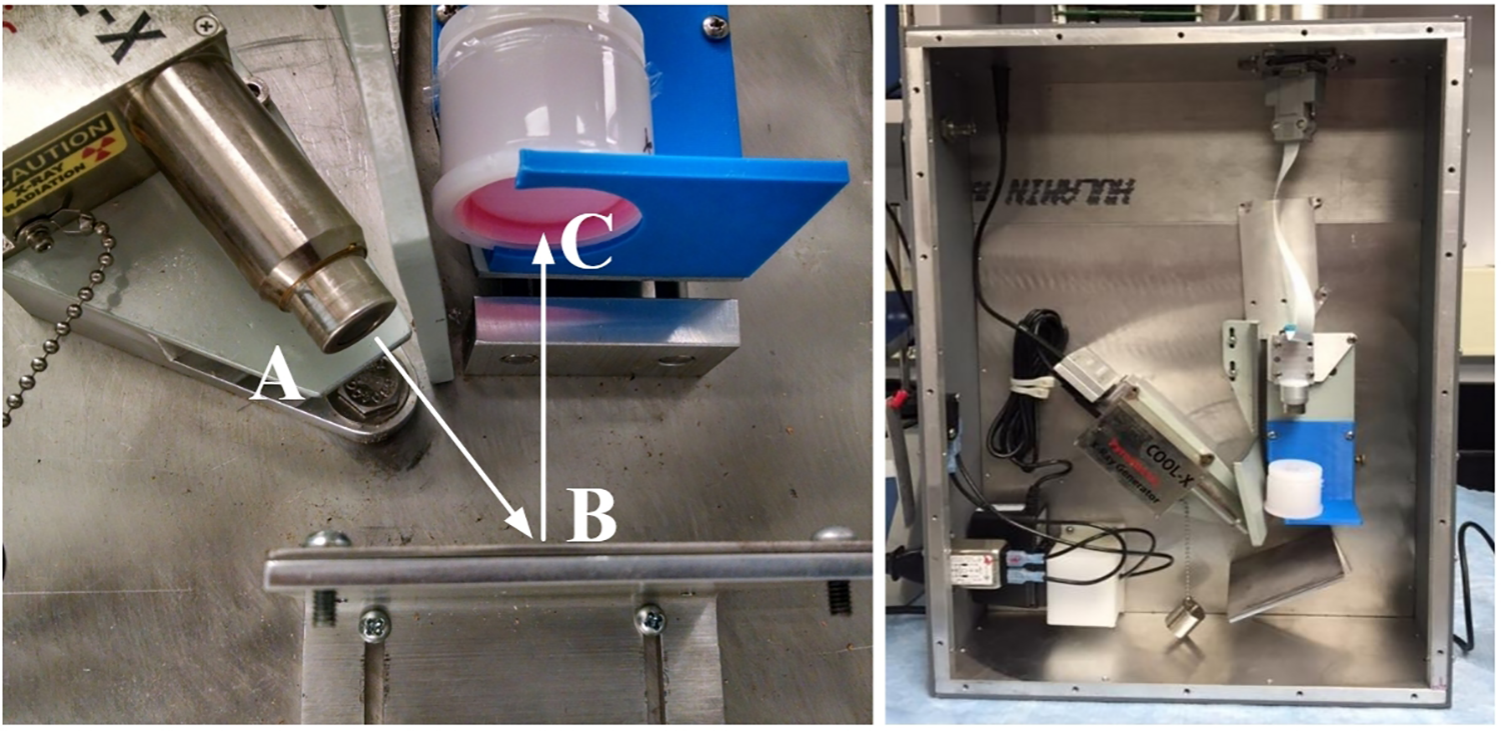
X-ray irradiator setup. The radiation source (A) targeted the cell culture by fluorescing off the metal plate (B) before irradiating the culture through the Mylar^®^ from the base of the cup (C).
